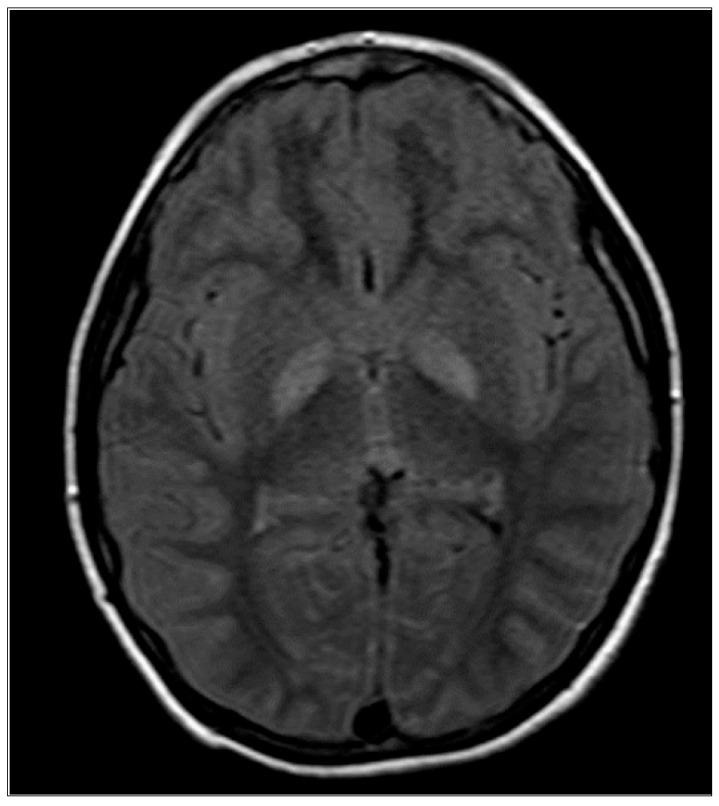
MRI brain scan of Case B. FLAIR sequencing found in MRI of Case B at eight years of age.
